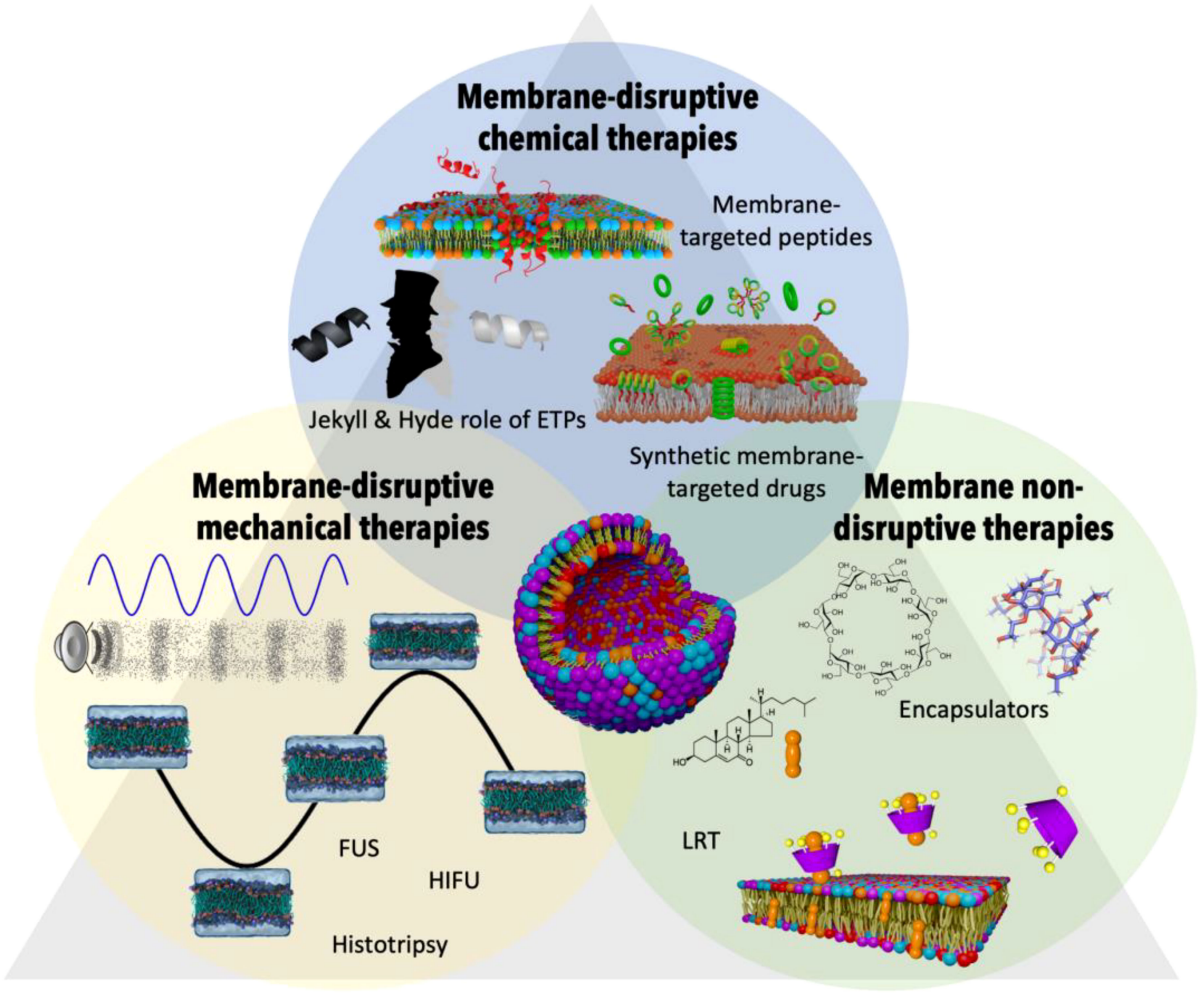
Therapeutic strategies targeting altered membrane lipids in the CAIn triangle. This figure offers a visual summary of various therapeutic interventions aimed at addressing the altered membrane compositions seen in conditions of cancer, infection, and aging. Membrane-disruptive chemical therapies: This group includes membrane-targeted peptides and synthetic membrane-targeted drugs that interact with and disrupt the membrane lipids of cancerous or otherwise diseased cells. The concept of the ‘Jekyll & Hyde role of ETPs’ is highlighted, noting the potential for both therapeutic and harmful effects of these peptides. Membrane-disruptive mechanical therapies: Depicted are techniques such as Focused Ultrasounds (FUS), High-Intensity Focused Ultrasounds (HIFU), and Histotripsy, which physically disrupt membrane integrity to target diseased cells, leveraging the mechanical forces to induce therapeutic effects. Membrane non-disruptive therapies: This section portrays encapsulator molecules that actively remove harmful lipids such as oxidized cholesterol from the membrane without disrupting its structure. Alongside, Lipid Replacement Therapy (LRT) is a complementary approach that seeks to restore healthy lipid composition, thereby aiding in the maintenance of membrane integrity and overall cellular function within the context of CAIn diseases.
